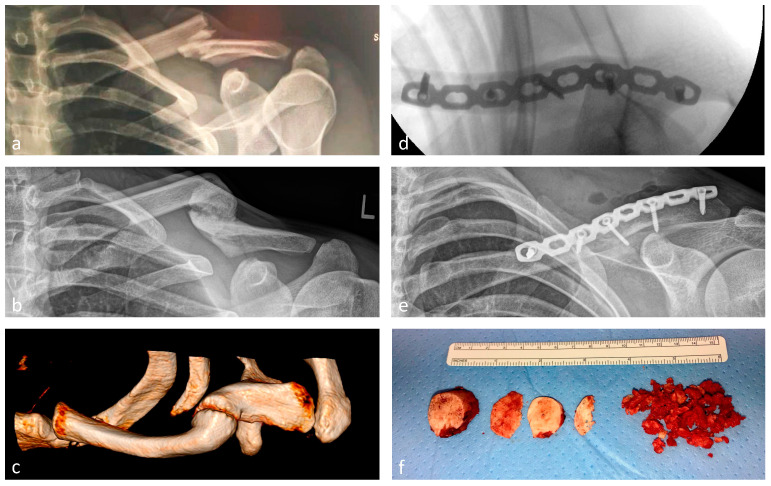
(a) Anterior–posterior radiograph of a fracture of a left clavicle shaft; (b) anterior–posterior radiograph of the developed symptomatic hypertrophic non-union after non-operative treatment; (c) 3D reconstruction via computer tomography; (d) intraoperative radiograph in axial direction after the resection of the non-union and internal fixation with an anatomically bent and twisted 3.5 mm LCP reconstruction plate combined with an interfragmentary lag screw; (e) postoperative anterior–posterior radiograph to demonstrate the adequate length of the clavicle and the anterior–superior placement of the plate osteosynthesis; (f) intraoperative picture of the resected hypertrophic non-union.
